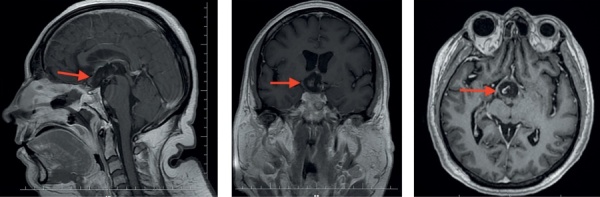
Рисунок 3. МРТ пациентки З. после операции. На фоне послеоперационных изменений визуализируются фрагменты капсулы опухоли в области III желудочка (указаны стрелкой).Figure 3. MRI of patient Z. after surgery. Against the  background of postoperative changes, fragments of the tumor capsule are visualized in the region of the third ventricle (indicated by an arrow).
